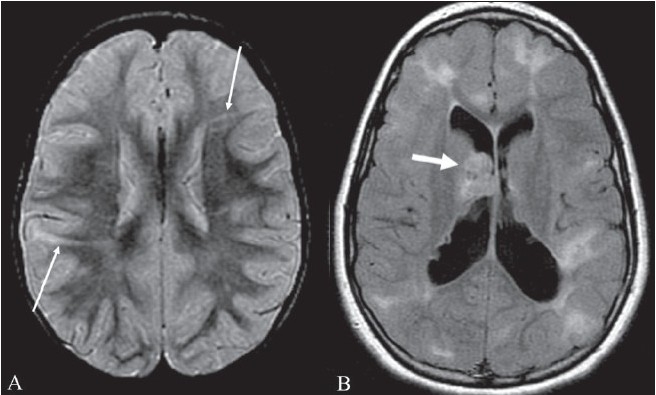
Radial band sign. Proton density-weighted axial image of the brain (A) in a patient with tuberous sclerosis shows hyperintense bands in the white matter radiating from the ventricular margin to the subcortical region (arrows). FLAIR axial MRI image (B) shows irregular hyperintense areas in the subcortical white matter suggestive of subcortical tubers. A large hyperintense nodule is seen in the region of the foramen of Monroe, representing a subependymal giant cell astrocytoma (thick arrow)
